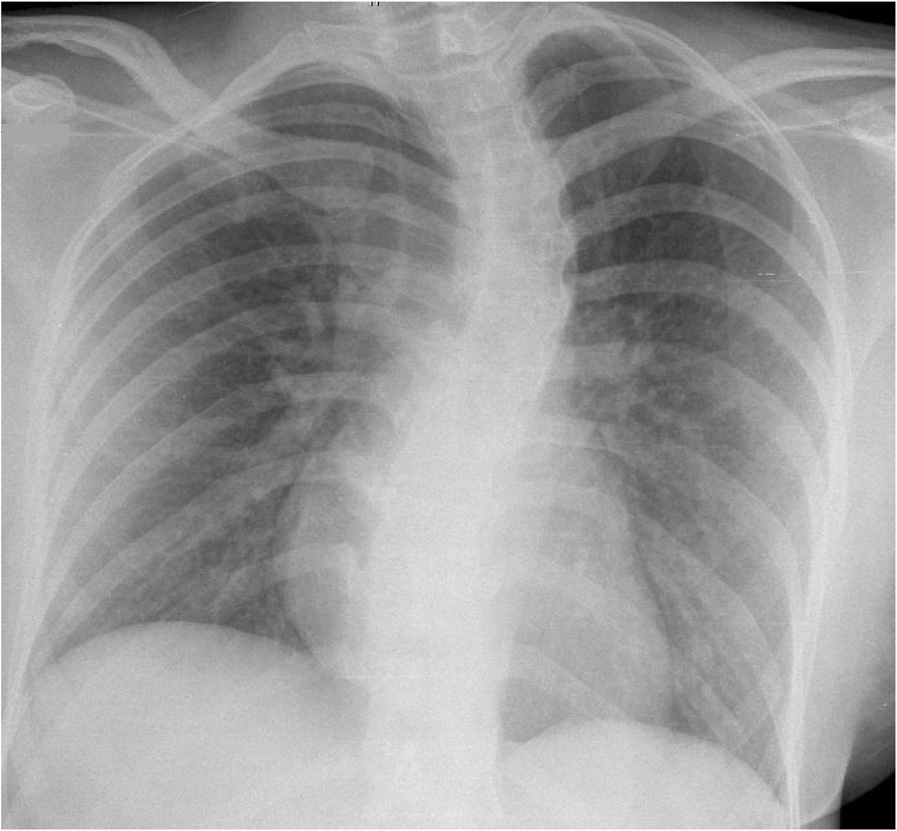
Chest X-ray taken at the first presentation showing milky opacifications in the basal parts of both lungs as well as a scoliosis. Courtesy of Prof. Kahn, Department of Imaging, University Hospital Leipzig.
